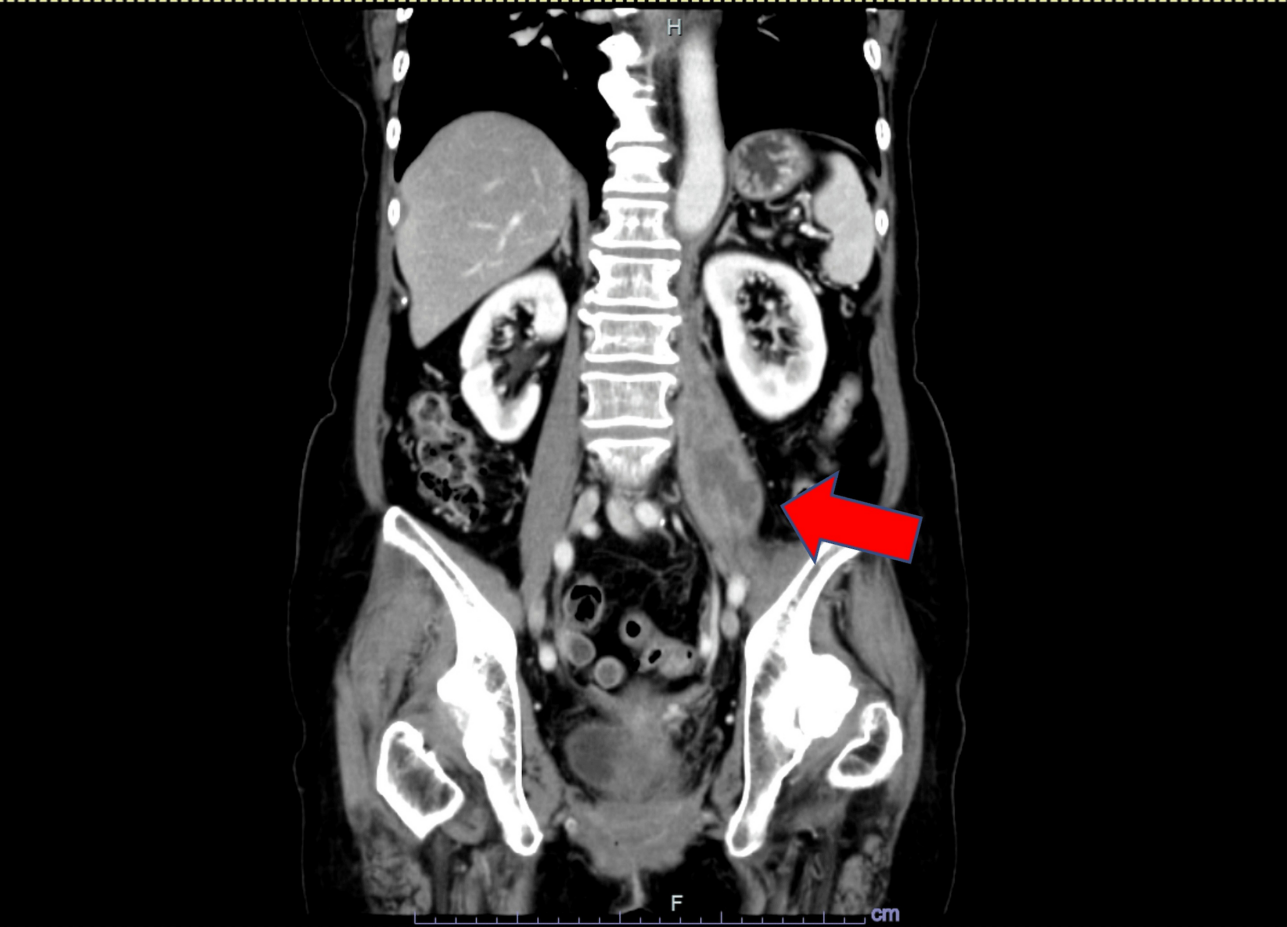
Coronal computed tomography findings. Low-density mass in the left psoas muscle with enhancing peripheral rim (arrow), measuring 67 × 32 mm.
